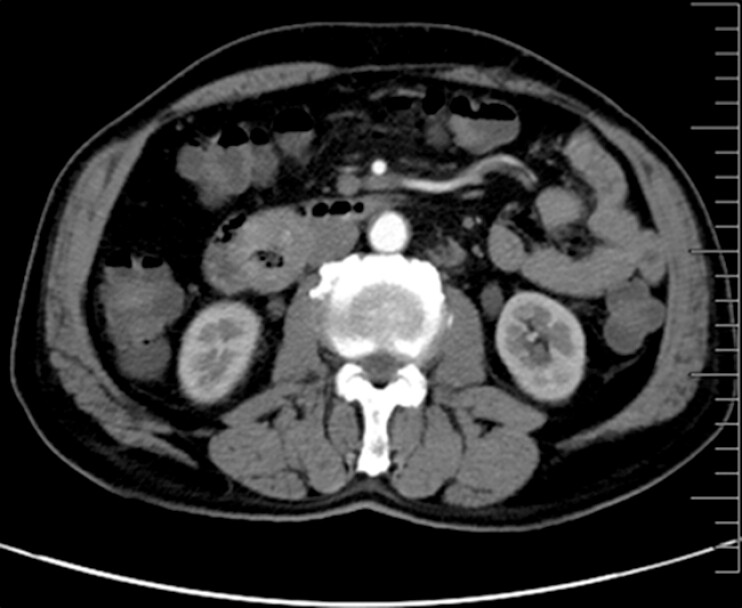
Computed tomography performed 4 months after treatment.
